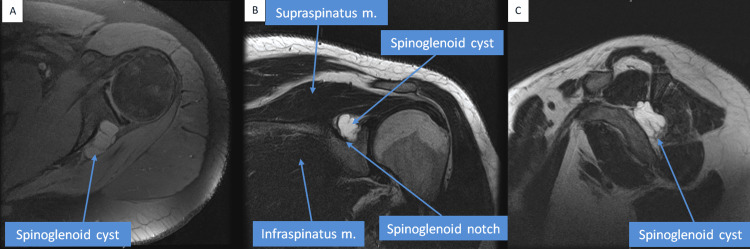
MRI of the patient Selected MRI sections show the presence of a multilobulated cystic-like structure seen extending from the supraspinatus notch reaching anteriorly to the infraspinatus muscle. The lesion measures about 3 x 2 x 3 cm and is consistent with a spinoglenoid ganglion cyst. A. Sagittal view. B. Coronal view. C. Axial view MRI: Magnetic Resonance Imaging
